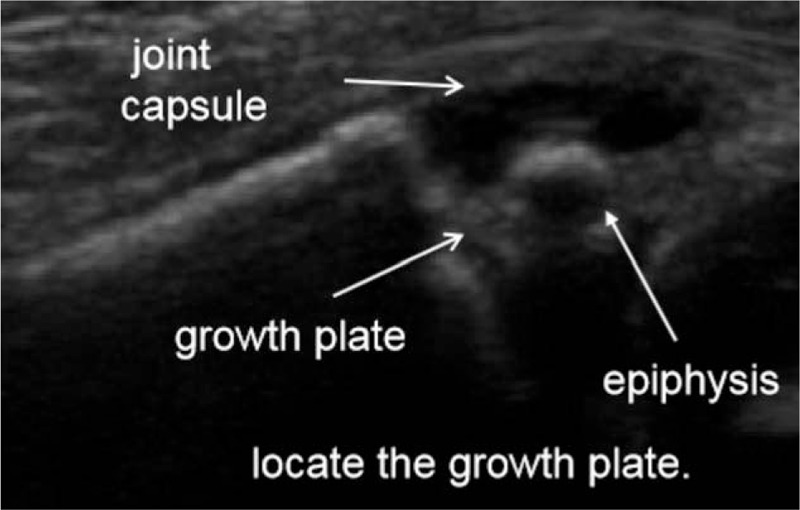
Ultrasound can be used to locate the growth plate.
